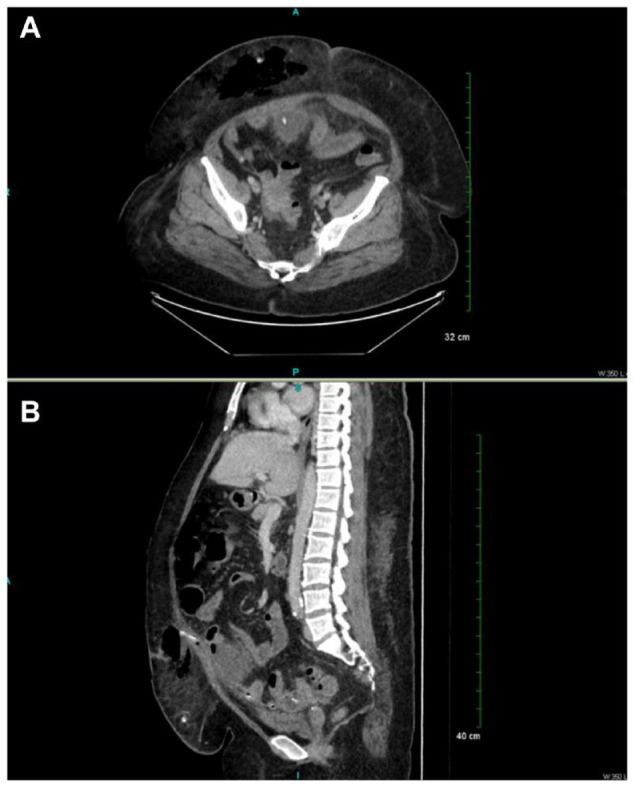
Abdominal computed tomography (CT) scan without contrast. (A) CT scan of the abdomen (axial view) demonstrates extensive organized subcutaneous and rectus muscle edema with extensive gas in the abdominal wall. (B) CT scan of the abdomen (sagittal view) demonstrates rim-enhancing air-fluid levels in mildly dilated and thickened small bowel, with mesenteric edema and fluid throughout the colon.
